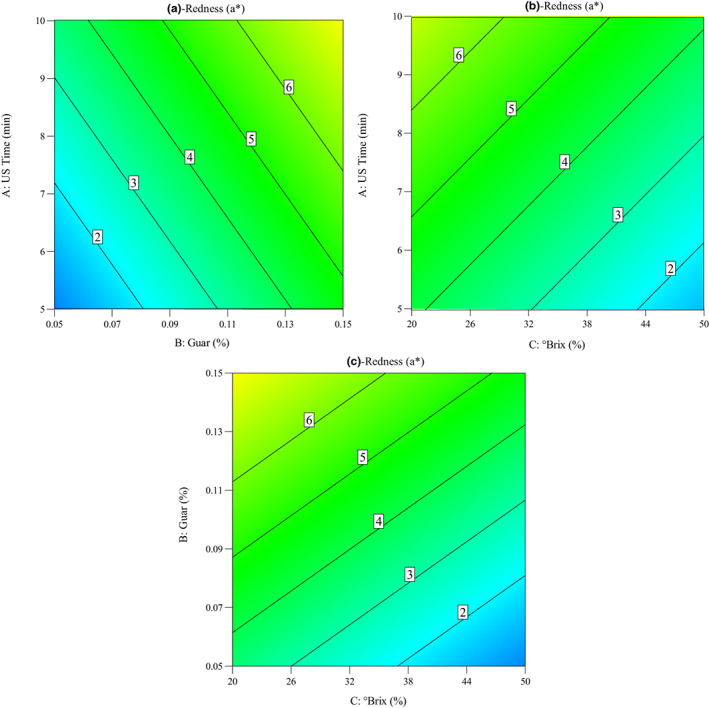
Contour plots for the impacts of osmotic dehydration parameters on the redness index of quince slices: (a) ultrasound time versus guar concentration, (b) ultrasound time versus °Brix, and (c) guar concentration versus °Brix.
